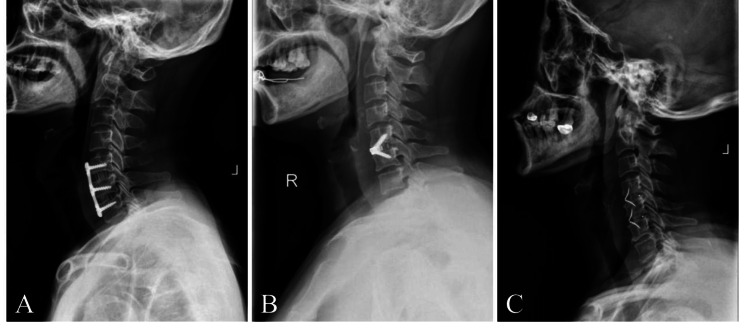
Postoperative lateral radiographs showing. (A) a patient with C5-6, C6-7 anterior cervical discectomy and fusion (ACDF) with a traditional titanium plate with cage, (B) a patient with C5-6ACDF with a Zero-p implant, and (C) a patient with C4-5, C5-6 ACDF with a ROI-C implant
